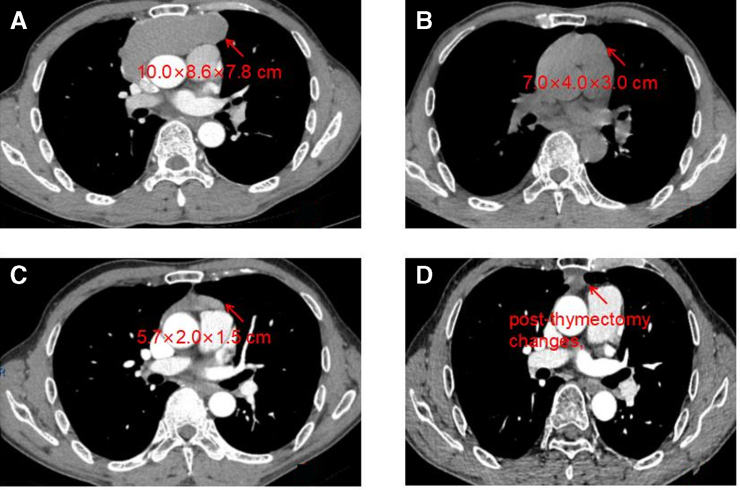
Serial contrast-enhanced CT images. (A) Baseline (July 2023): large anterior mediastinal mass (10.0 × 8.6 × 7.8 cm) with abutment of the left innominate vein/right atrial appendage (red arrow). (B) After 1 cycle of CAP: marked regression to ~7.0 × 4.0 × 3.0 cm (red arrow). (C) After 2 cycles: further reduction to 5.7 × 2.0 × 1.5 cm residual strand/fibrosis (red arrow). (D) Postoperative (3 months): postsurgical changes at the thymectomy site; no local recurrence. CAP = cyclophosphamide, doxorubicin, cisplatin, CT = computed tomography.
